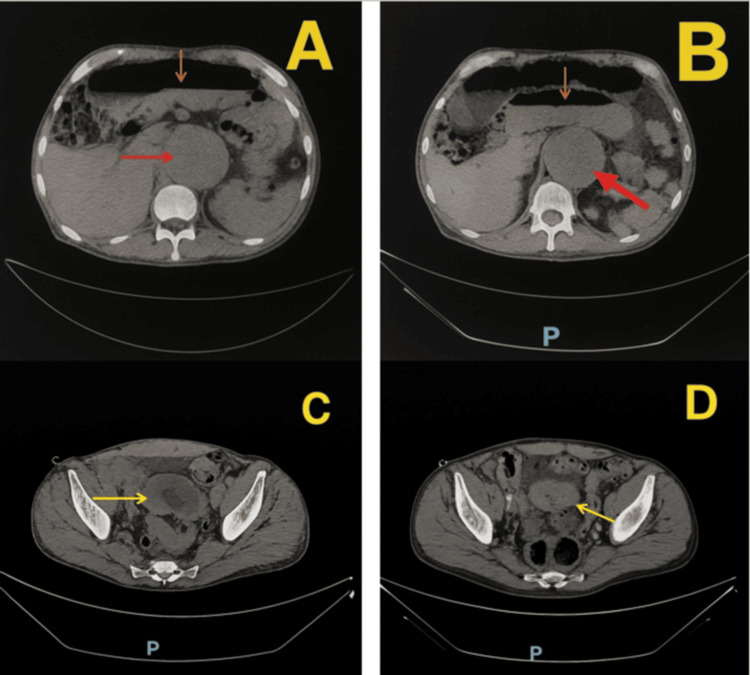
Abdominopelvic CT findings A: abdominal aortic aneurysm (red arrow), with dilated proximal duodenum with air-fluid levels (orange arrow); B: dilated distal duodenum and proximal jejunum with air-fluid levels (orange arrow) and an abdominal aortic aneurysm (red arrow); C: distal ileal-ileal small bowel intussusception with classic “target sign" appearance (yellow arrow); D: bowel narrowing distal to intussusception with hyperintensity (yellow arrow).
